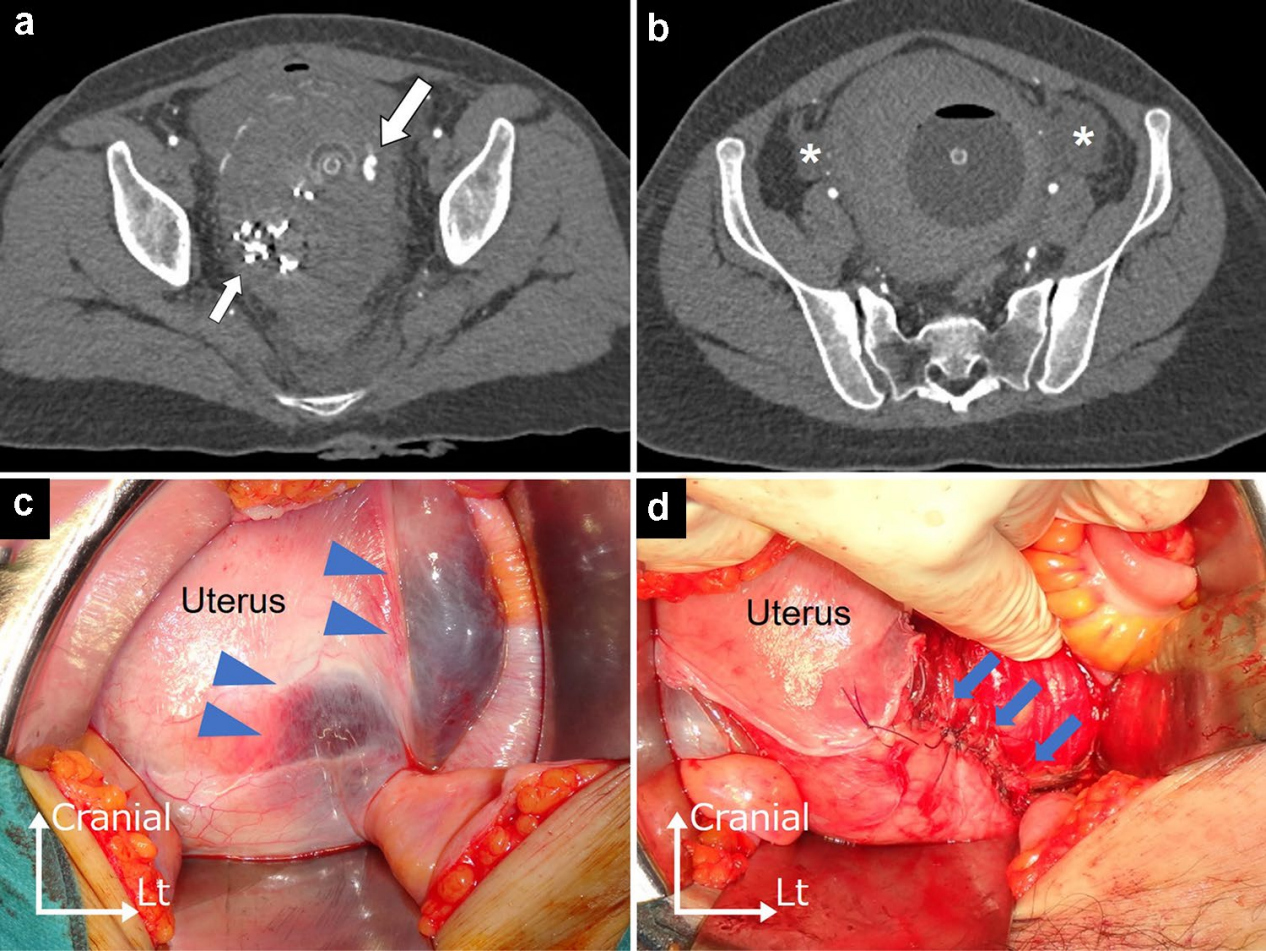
Patient 2: a, b contrast-enhanced computed tomography images obtained during the arterial phase demonstrating extravasation (large arrow). The small arrow indicates iodoform gauze. A hematoma (asterisks) can be seen in the broad ligament. An intrauterine balloon tamponade device was placed. c Hematoma spreading in the broad ligament (blue arrowheads). d The broad ligament was incised, and an 8 cm laceration on the left lower uterus was sutured (blue arrows)
